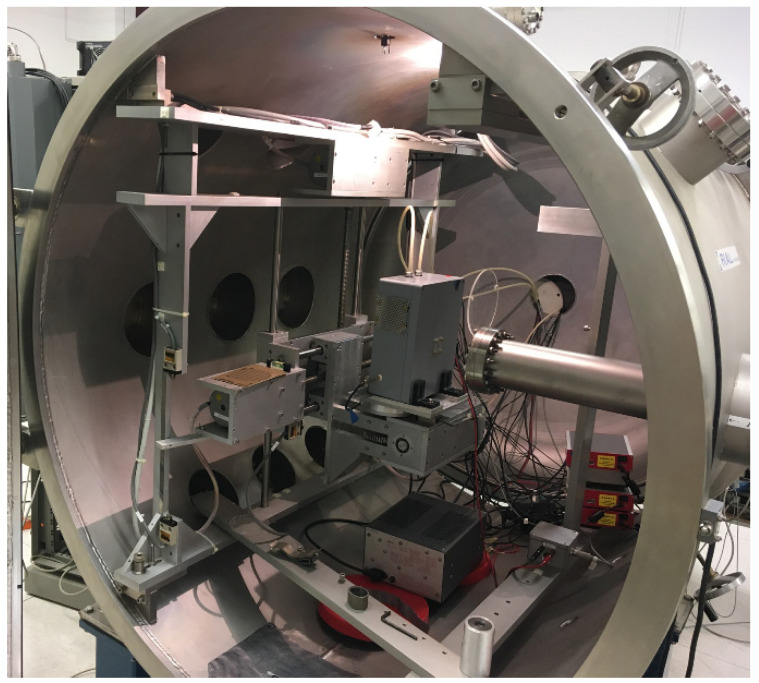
An overview of the experimental setup used for high-flux X-ray measurements at the Livio Scarsi X-ray facility. The CZT pixel detectors (enclosed in the grey rectangular box, together with the preamplifier PIXIE ASIC) were irradiated with Mo target X-rays (the tube on the right side). The red boxes, on the bottom right side, are the digitizers of the digital pulse processing electronics. The detector box was mounted on a micro-translator system, which can be moved in *x*, *y*, and *z* directions with a precision of 10 μm.
